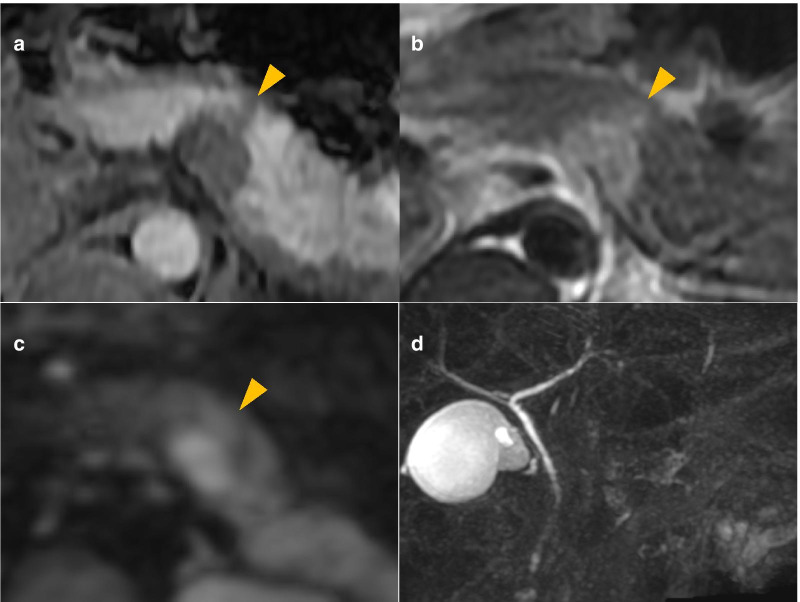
Magnetic resonance imaging revealed a mass (triangles) exhibiting hypointensity on T1-weighted images (a), hyperintensity on T2-weighted images b and diffusion-weighted images c at the pancreas body and tail. Magnetic resonance cholangiopancreatography showed no dilation of the pancreatic duct (d)
